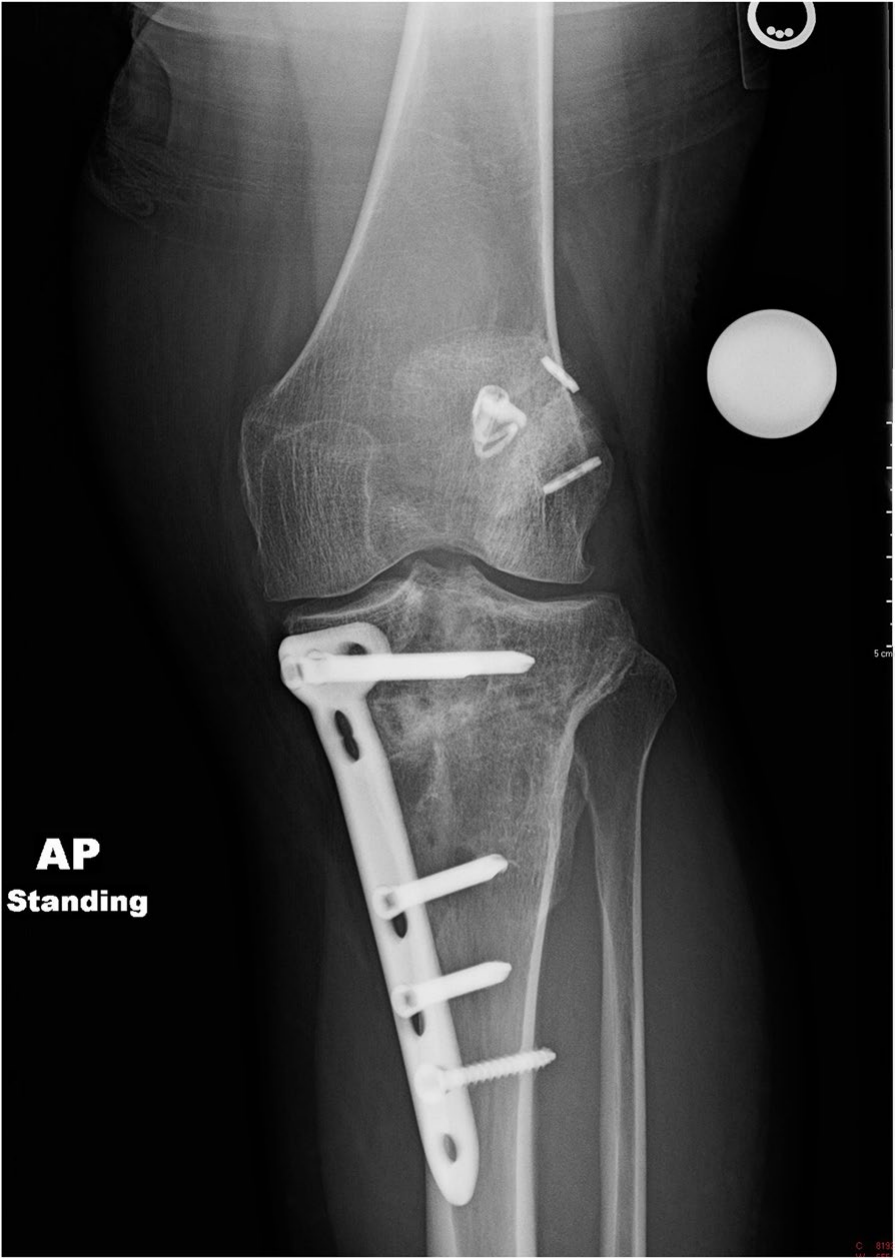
An AP radiograph showing the healed osteotomy
